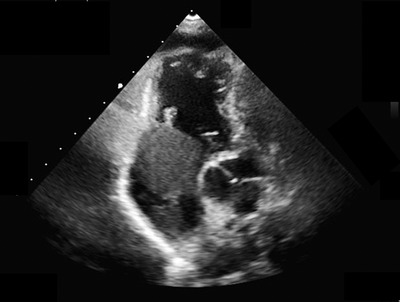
Transthoracic echocardiography revealed tumor filling in the right atrium
